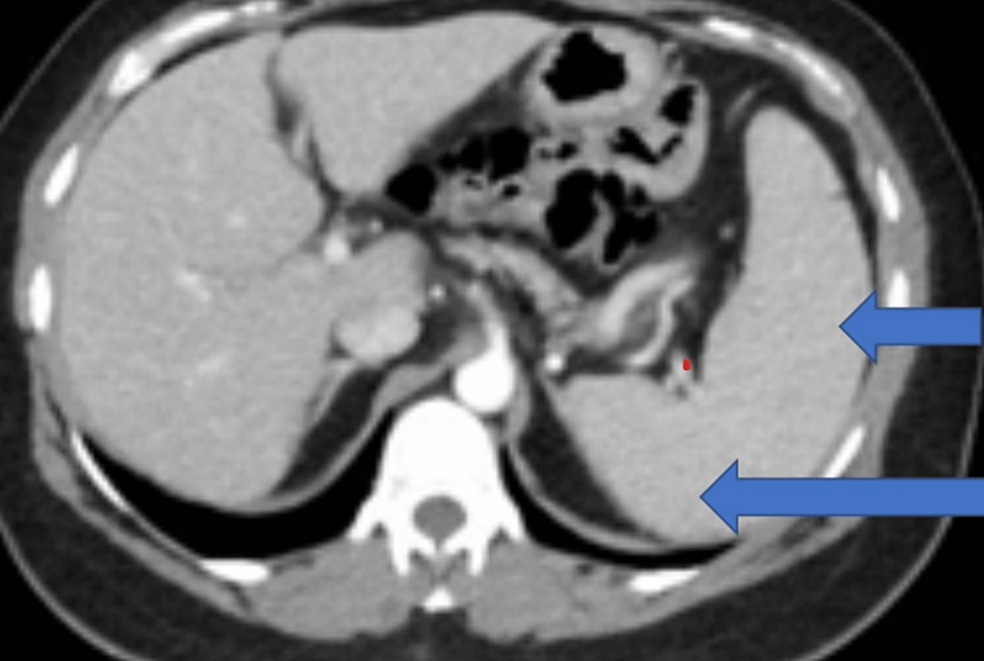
CECT abdomen showing splenomegaly (blue arrows) CECT: contrast-enhanced computed tomography
